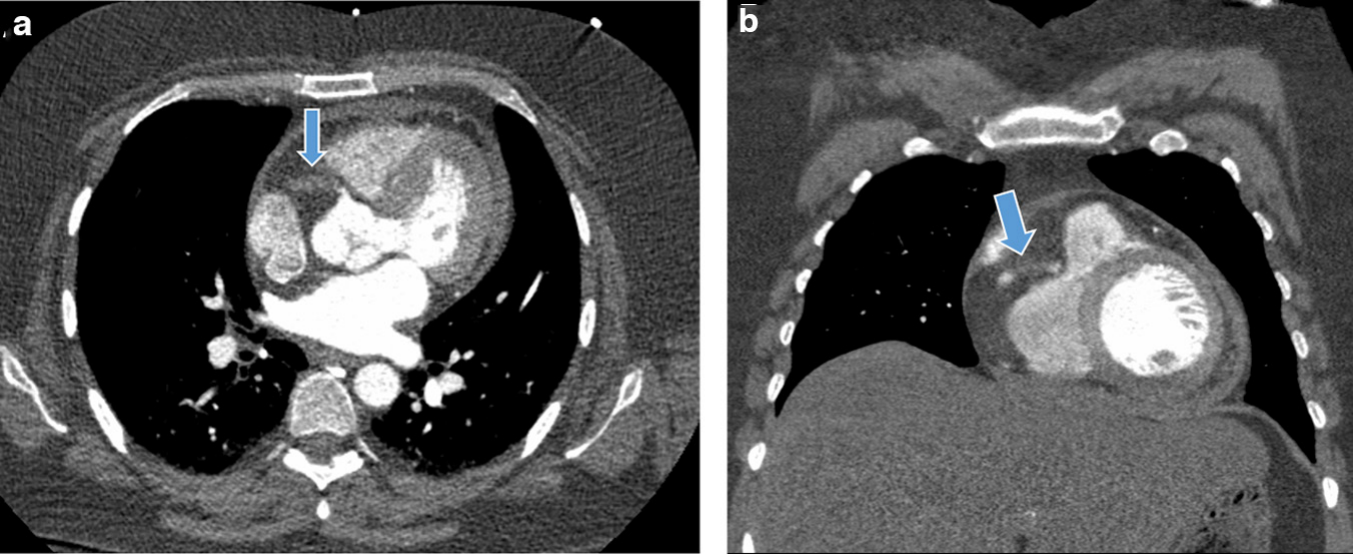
A and B. Contrast-enhanced CT images initially obtained for chest pain 5 days prior. (A.) Axial contrast-media-enhanced image of the chest demonstrating an abrupt 2 cm occlusion of the proximal right coronary artery. (B) Coronal reformat image better demonstrating the occluded segment of the right coronary artery. Normal contrast flow is seen just proximal and distal to this.
